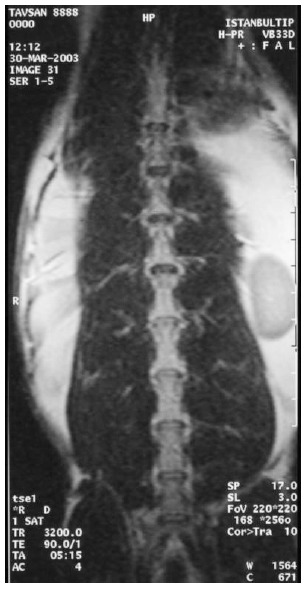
Discs after fibroblast injection on MRI.
